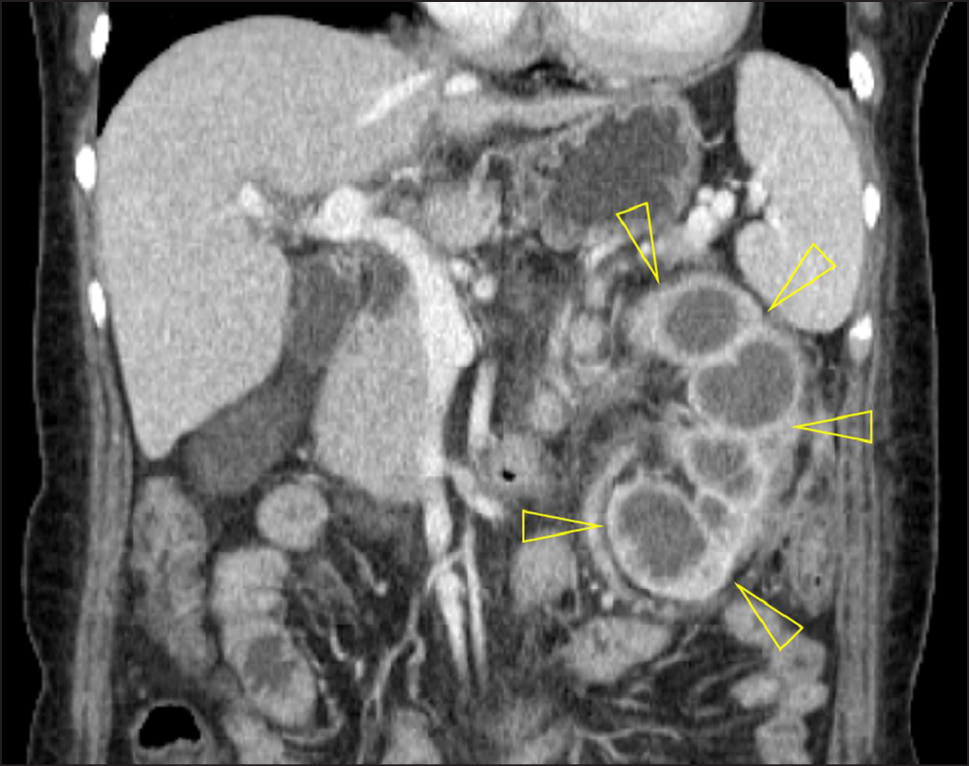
Coronal reformatted contrast-enhanced CT image shows an enlarged left kidney with surrounding inflammatory changes. The dilated renal calyces are similar to the footprint of a bear (open arrowheads).
